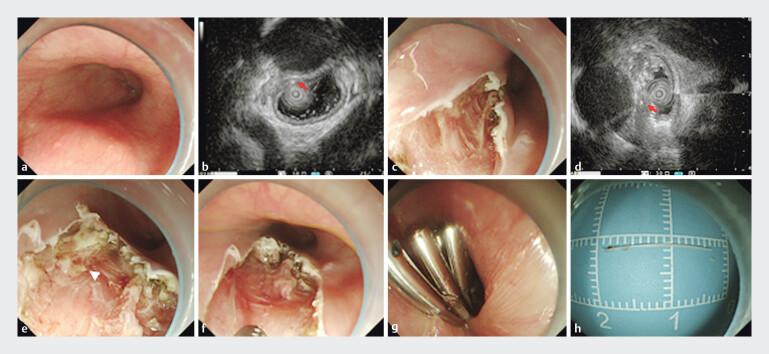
Endoscopic ultrasound (EUS)-guided endoscopic submucosal dissection to remove a fishbone embedded adjacent to the thoracic aorta.
